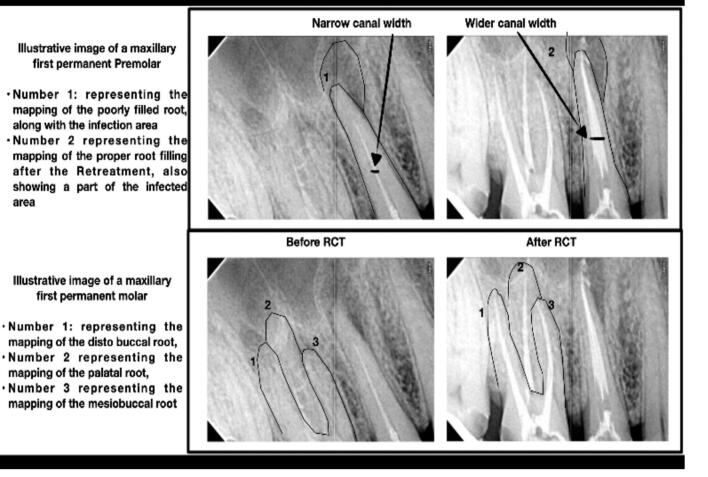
X-ray image of root canal of a patient before and after treatment with F1.
